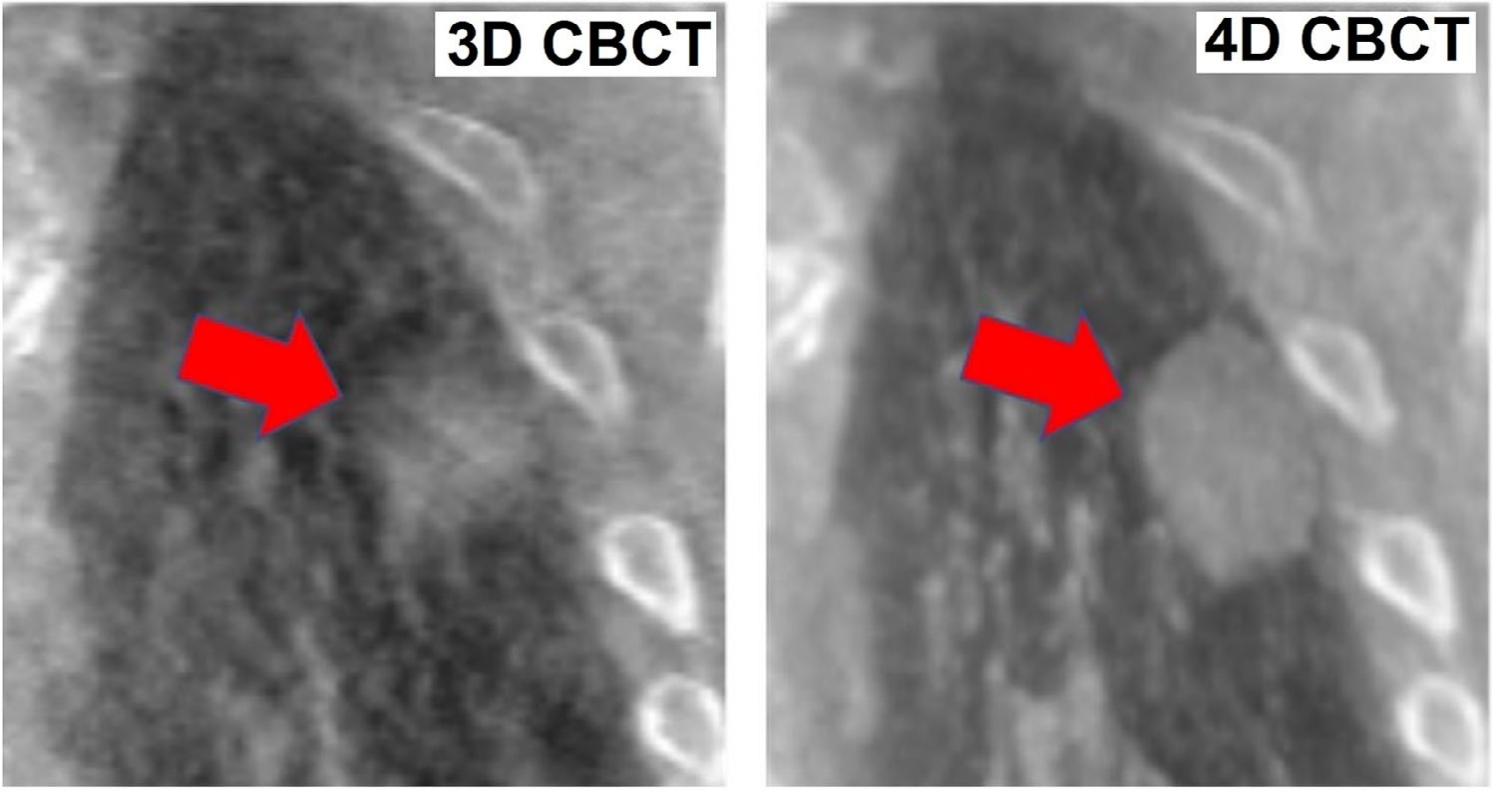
Sample subject for whom the difference between 4D CBCT and 3D CBCT was more pronounced. The EVM difference was 2.2 mm, and the degree of motion was 1.5 cm (for a 2 cm target). 3D CBCT, three‐dimensional cone‐beam CT; 4D CBCT, Four‐dimensional cone‐beam CT; EVM, edge visualization metric.
